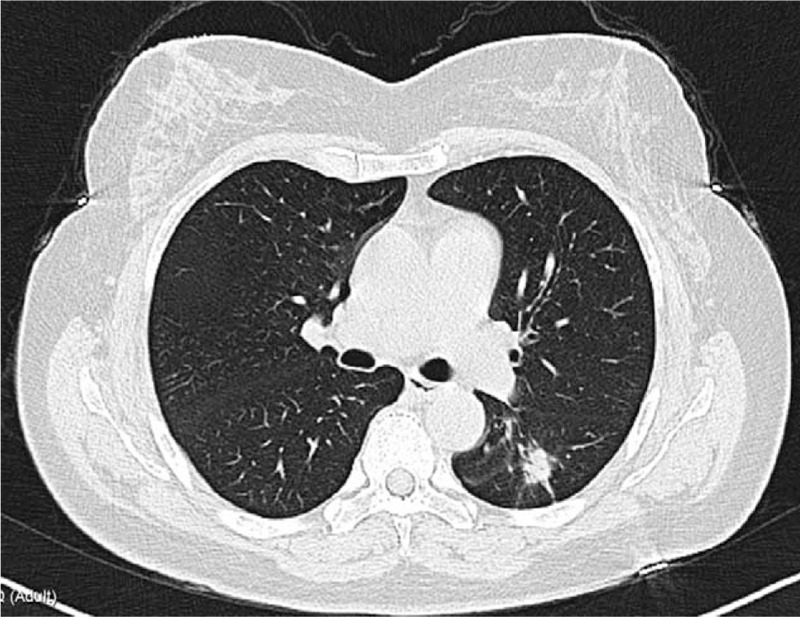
Chest computed tomography scan of this patient detected a ground-glass nodule (2 × 1.5 × 1.5 cm) on the left inferior lobe.
